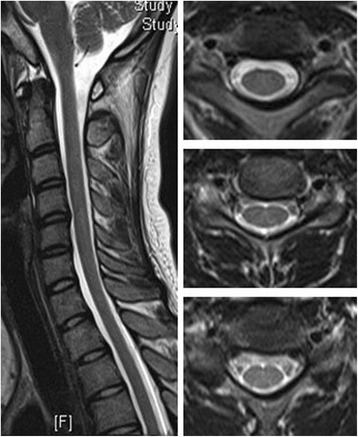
Follow-up cervical spinal cord of MRI revealed the resolution of the previously noted lesions of inverted V-sign within the posterior columns
